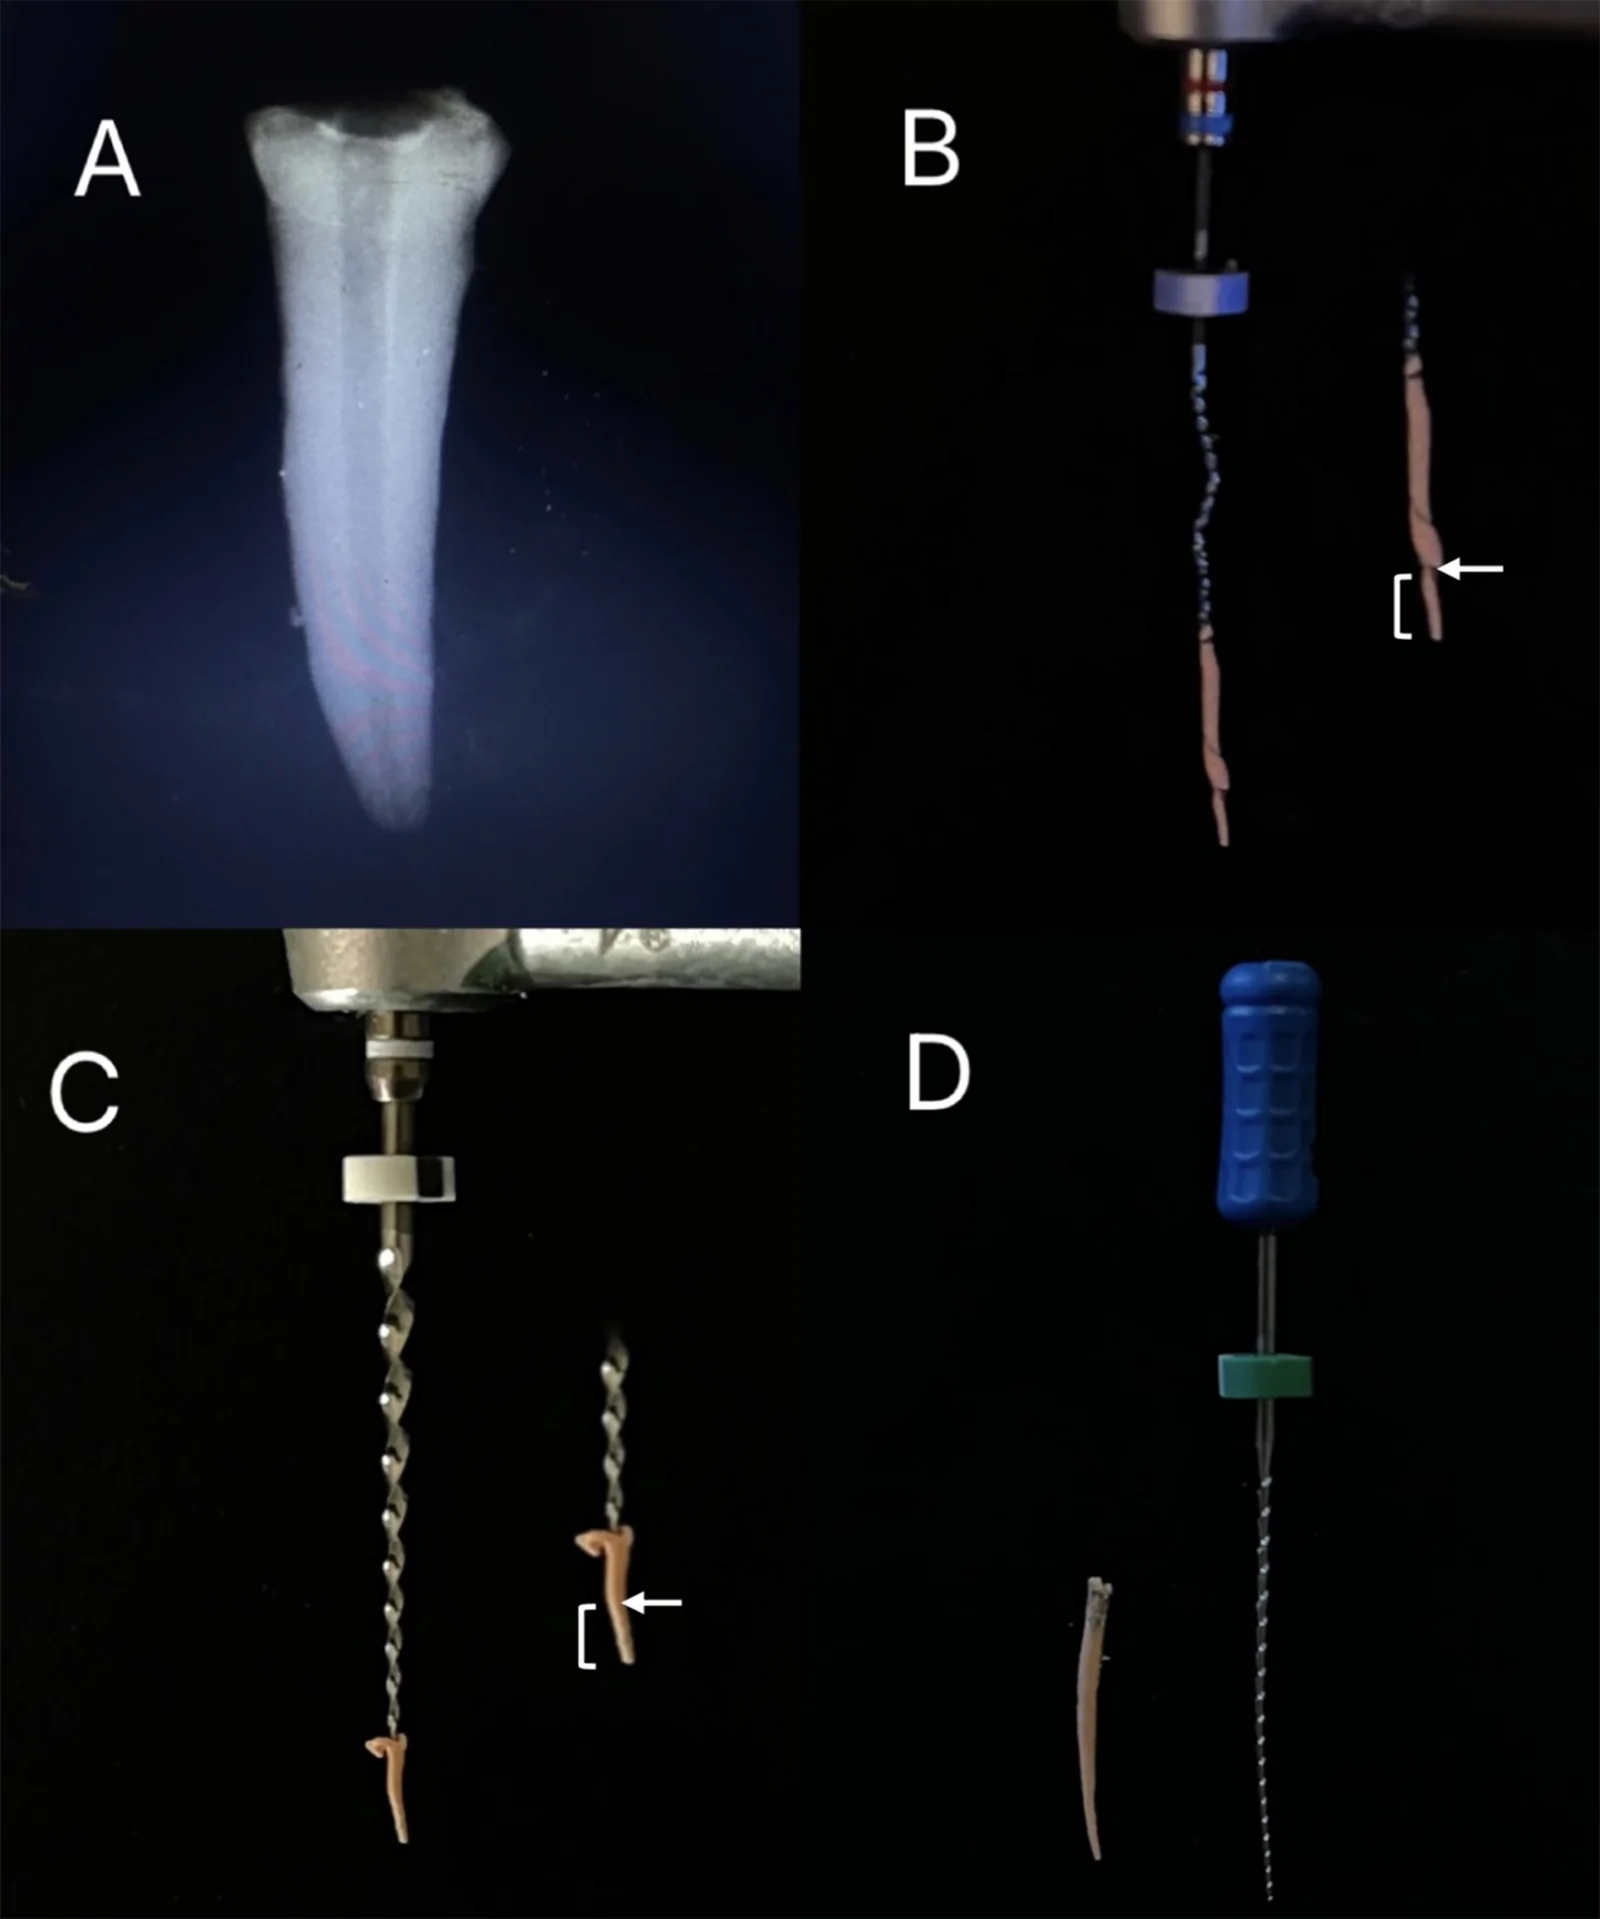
A Radiograph of the tooth after complete removal of overextended filling material, and (B) The XPER system after removal of the overextended gutta-percha. C The PTUR system after removal of the overextended gutta-percha. D The H-files system after removal of the overextended gutta-percha. (The arrows showing where the files end). (The square brackets show the 3 mm of gutta-percha which was overextended beyond the apex)
